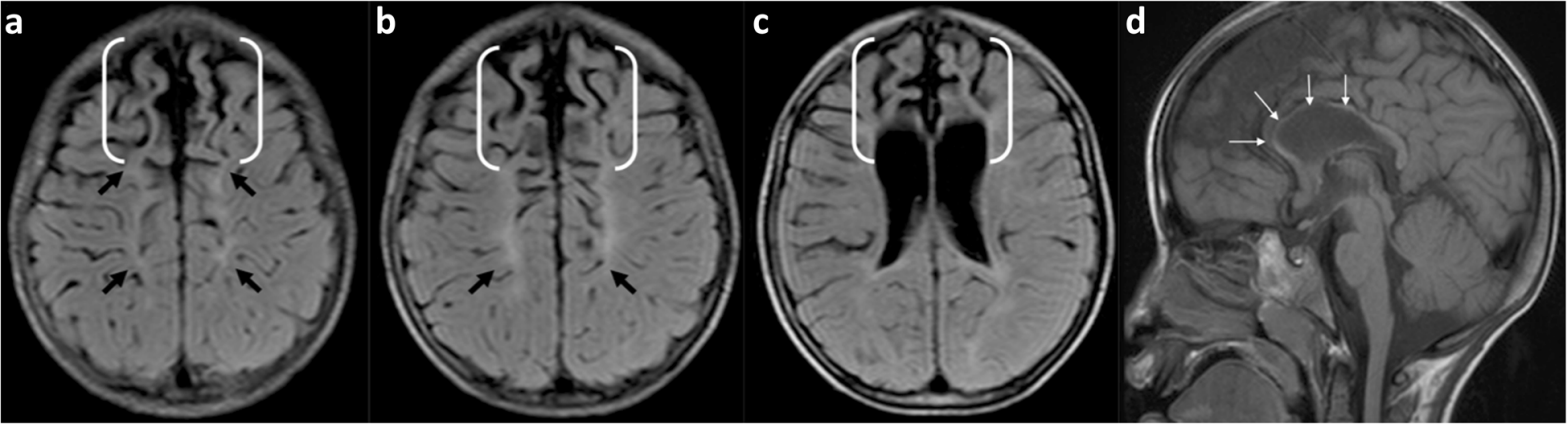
a–d An 8-year and 10-month-old boy on sequential axial FLAIR images from superior to inferior (a–c), demonstrating the bilateral, symmetric, predominantly anterior inter-vascular watershed involvement, manifesting as frontal atrophy and underlying signal abnormality in continuity with the parasagittal volume loss and signal abnormality (black arrows). There is an expansion of the inter-hemispheric fissure anteriorly and prominent, deep, superior frontal sulci bilaterally (white brackets). The sagittal T1-weighted midline image (d) demonstrates associated corpus callosum volume loss predominantly affecting the rostrum, genu, and anterior body (thin arrows)
